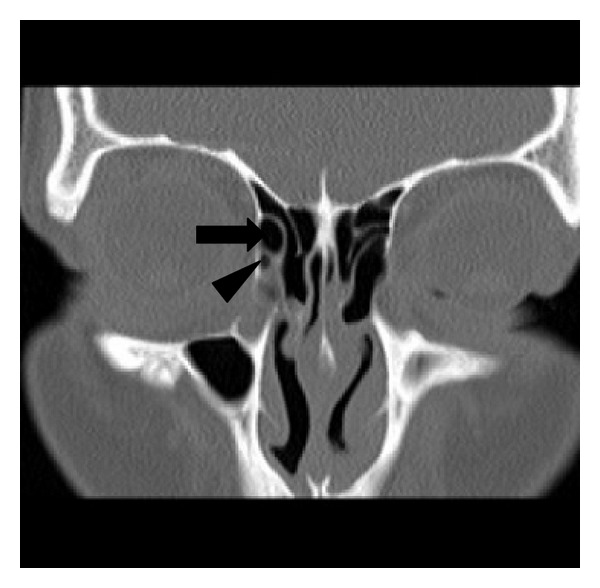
Type I frontal cell and agger nasi cell. A coronal CT scan showing a right-sided type I frontal cell (arrow) above an agger nasi cell (arrow head).
